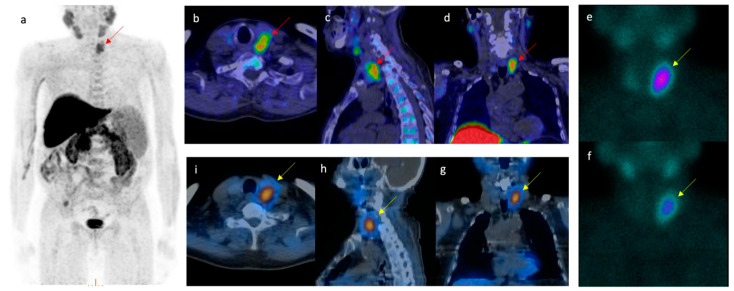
The clinical case of a 72-year-old man who underwent [^18^F]FCH PET/CT (a–d) revealed a focal [^18^F]FCH uptake posteriorly to the left thyroid lobe (red arrows), of probable parathyroid origin. The patient was directed to laboratory tests that showed a normal level of free triiodothyronine (FT3) (2.94 pg/mL, range 2.20–4.20) and free thyroxine (FT4) (12.4 pg/mL, range 8.10–17.1) as well as a normal thyroid-stimulating hormone (FSH) (1.34 mUI/L, range 0.3–3.6) but elevated PTH (761 pg/mL, range 14–72) and calcium levels (11.1 mg/dL, range 8.5–10.1). Consequently, the patient underwent [^99m^Tc]Tc-MIBI parathyroid scintigraphy (f–i) according to a dual-phase protocol (e,f) integrated with single photon emission computed tomography/computed tomography (SPECT/CT) (g–i). [^99m^Tc]Tc-MIBI identified the same focal area of increased uptake detected by [^18^F]FCH PET/CT (yellow arrows). Following the surgical removal, the pathology examination confirmed the presence of an atypical parathyroid adenoma (Ki67 < 10%) with a post-surgical normalization of PTH and calcium levels. Based on the scientific evidence that proves the superiority of [^18^F]FCH PET/CT compared with [^99m^Tc]Tc-MIBI in hyperparathyroidism, the patient could have avoided other diagnostic investigations, further radiation exposure and spared time for the cure.
